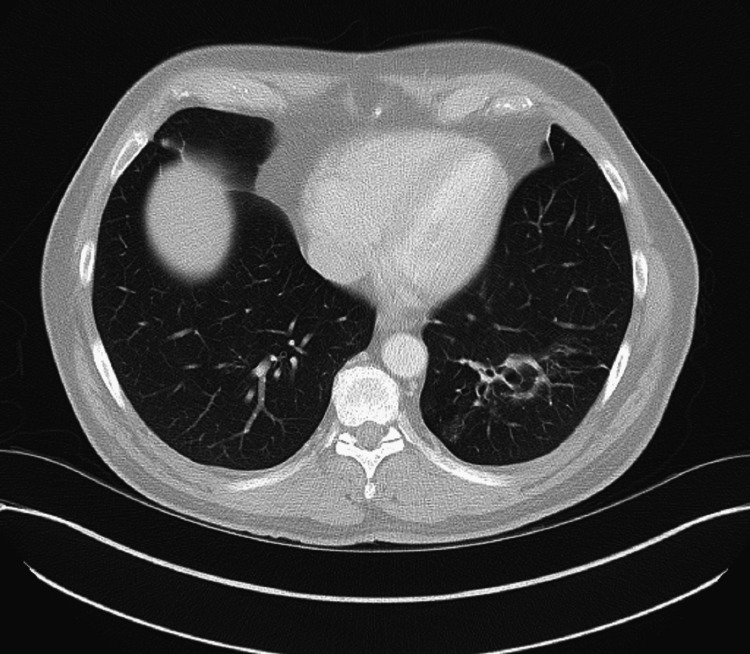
Our patient’s thoracic CT which showed shadowing and bronchiectasis in the left lower lung. Signed consent was obtained from the patient prior to using the image in the case report.
